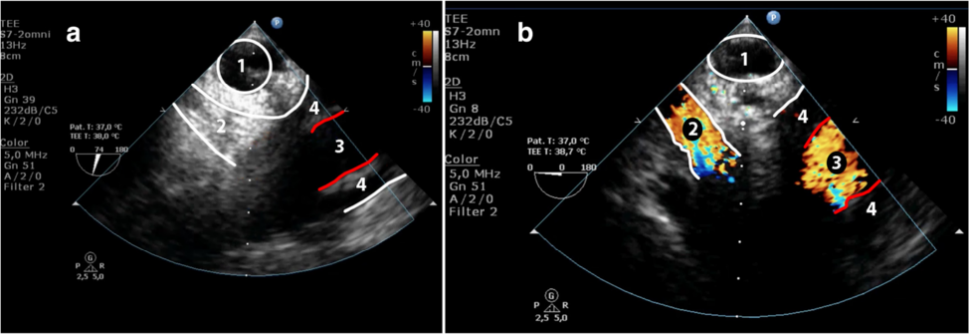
Modified TEE aortic arch short axis view. Panel (a) Plain 2D image showing a dissection starting in the proximal aortic arch, which continued distally into the descending aorta. The intimal tear did not progress into the innominate artery. Panel (b) Similar image with color-Doppler showing flow through the true aortic lumen, and a normal perfusion of the innominate artery. 1 = Trachea with inflated endotracheal balloon; 2 = Innominate artery; 3 = True lumen; 4 = False lumen; red lines delineate the intimal tear
